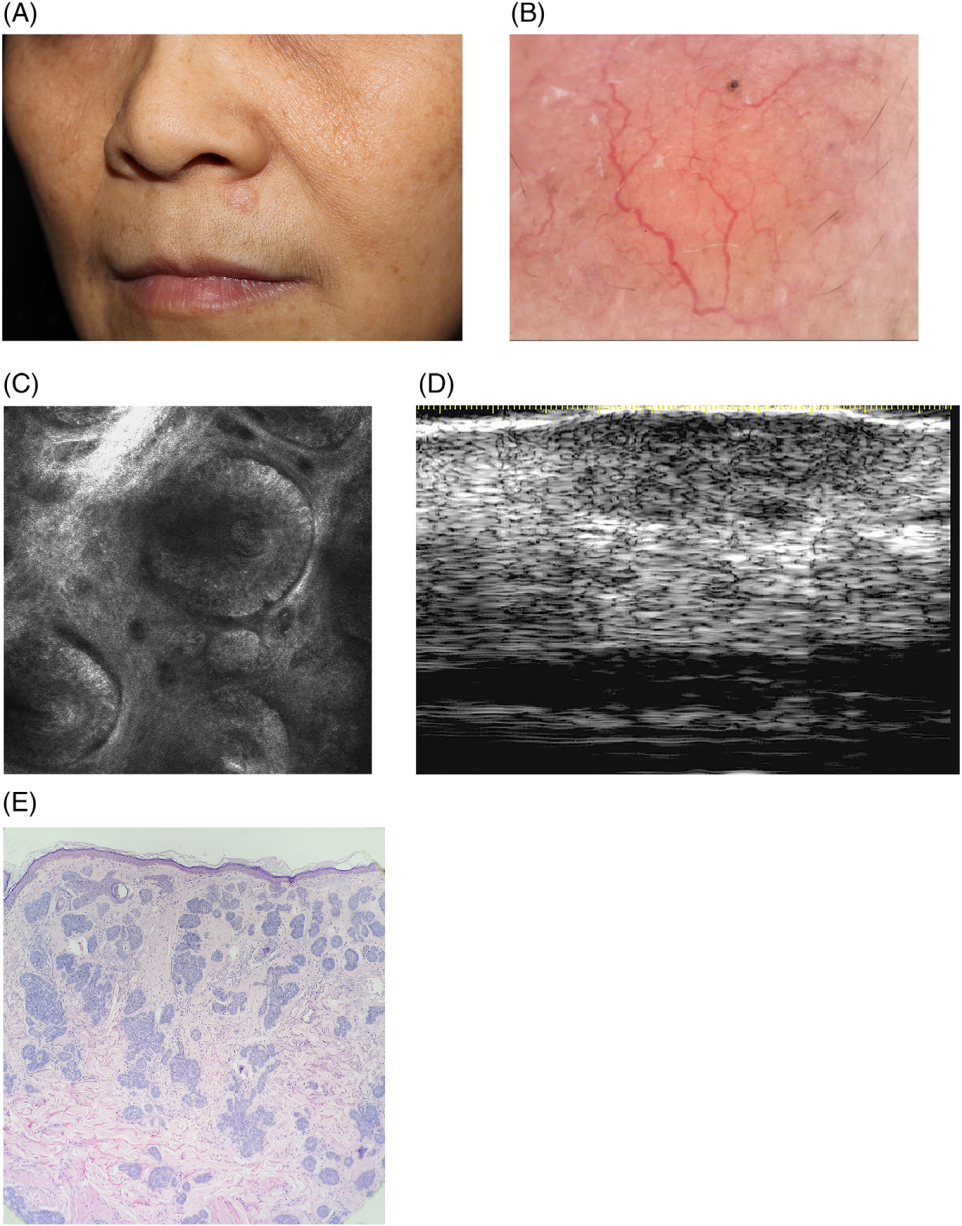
(A) A single skin‐colored nodule on the upper lip. (B) Dermoscopy: arborizing vessels with whitish structures on the pink background (×50 magnification). (C) RCM: bright tumor islands with peripheral palisading, cleft‐like dark spaces, and dilated canalicular vessels in the dermis (0.5 × 0.5 μm magnification). (D) Ultrasound: an irregular hypoechoic dermal and hypodermal structure (20 MHz) (E) Strands and nests of basaloid cells surrounding dense and sclerotic fibrous stroma in the dermis and subcutaneous tissue (HE ×100)
